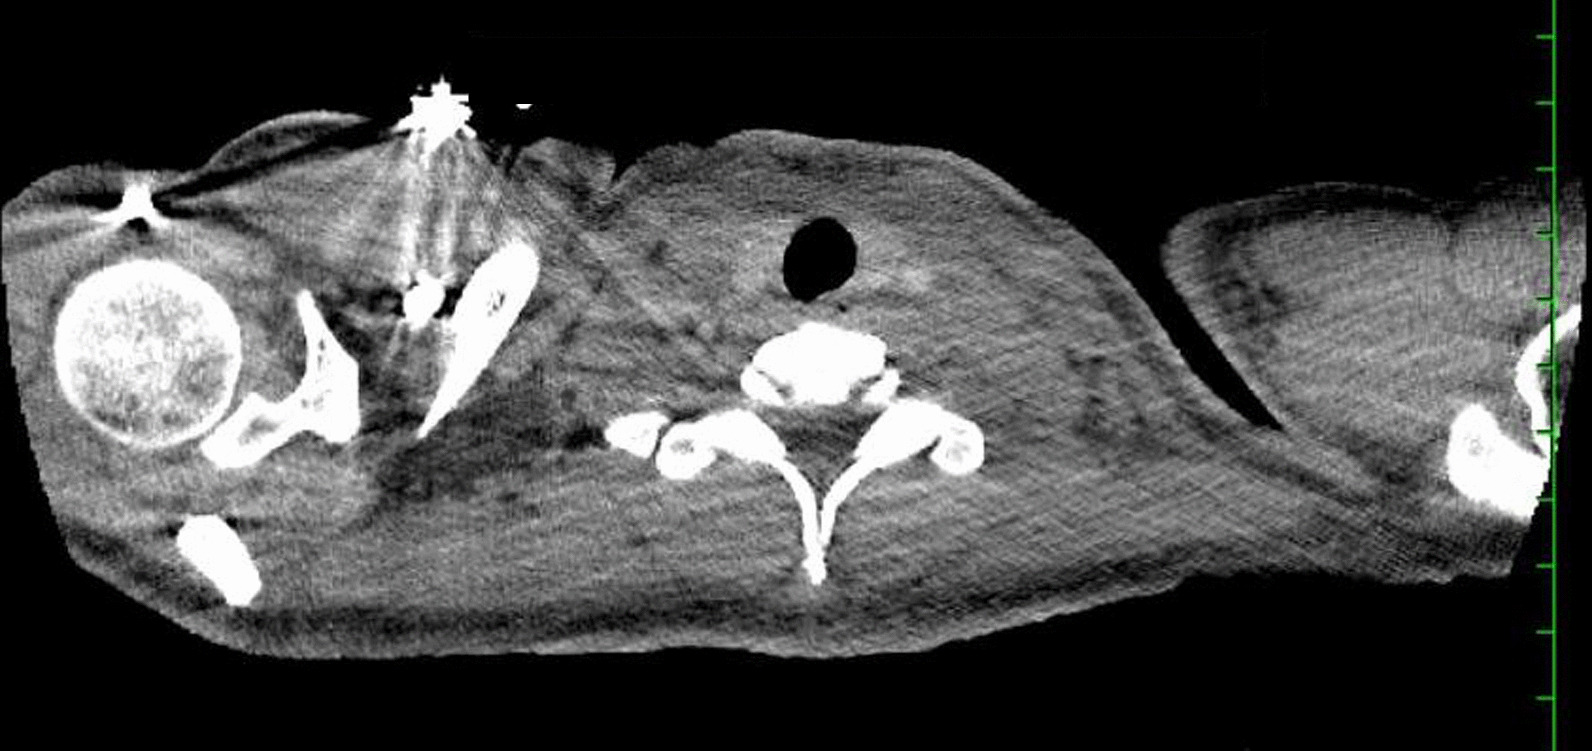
The diffuse lymphadenopathy noted on CT abdomen/pelvis from admission
